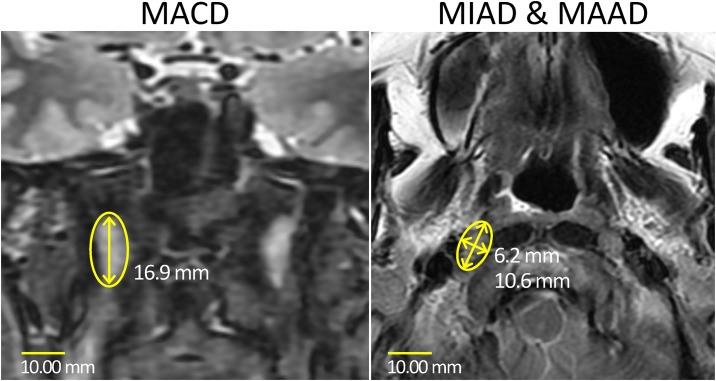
Measuring the three diameters of a right lateral retropharyngeal node. This image was obtained from a 48-year-old NPC woman with a lateral retropharyngeal lymph node (yellow circled) on a T2-weighted MRI. (a) In axial plane, the MAAD (10.6 mm) should be measured first, and the MIAD (6.2 mm) should then be measured in the perpendicular direction. (b) Coronal plane of image showing that the MACD (16.9 mm) of the node can be measured using an electronic caliper easily. NPC, nasopharyngeal cancer; MIAD, minimal axial diameter; MAAD, maximal axial diameter; MACD, maximal coronal diameter.
